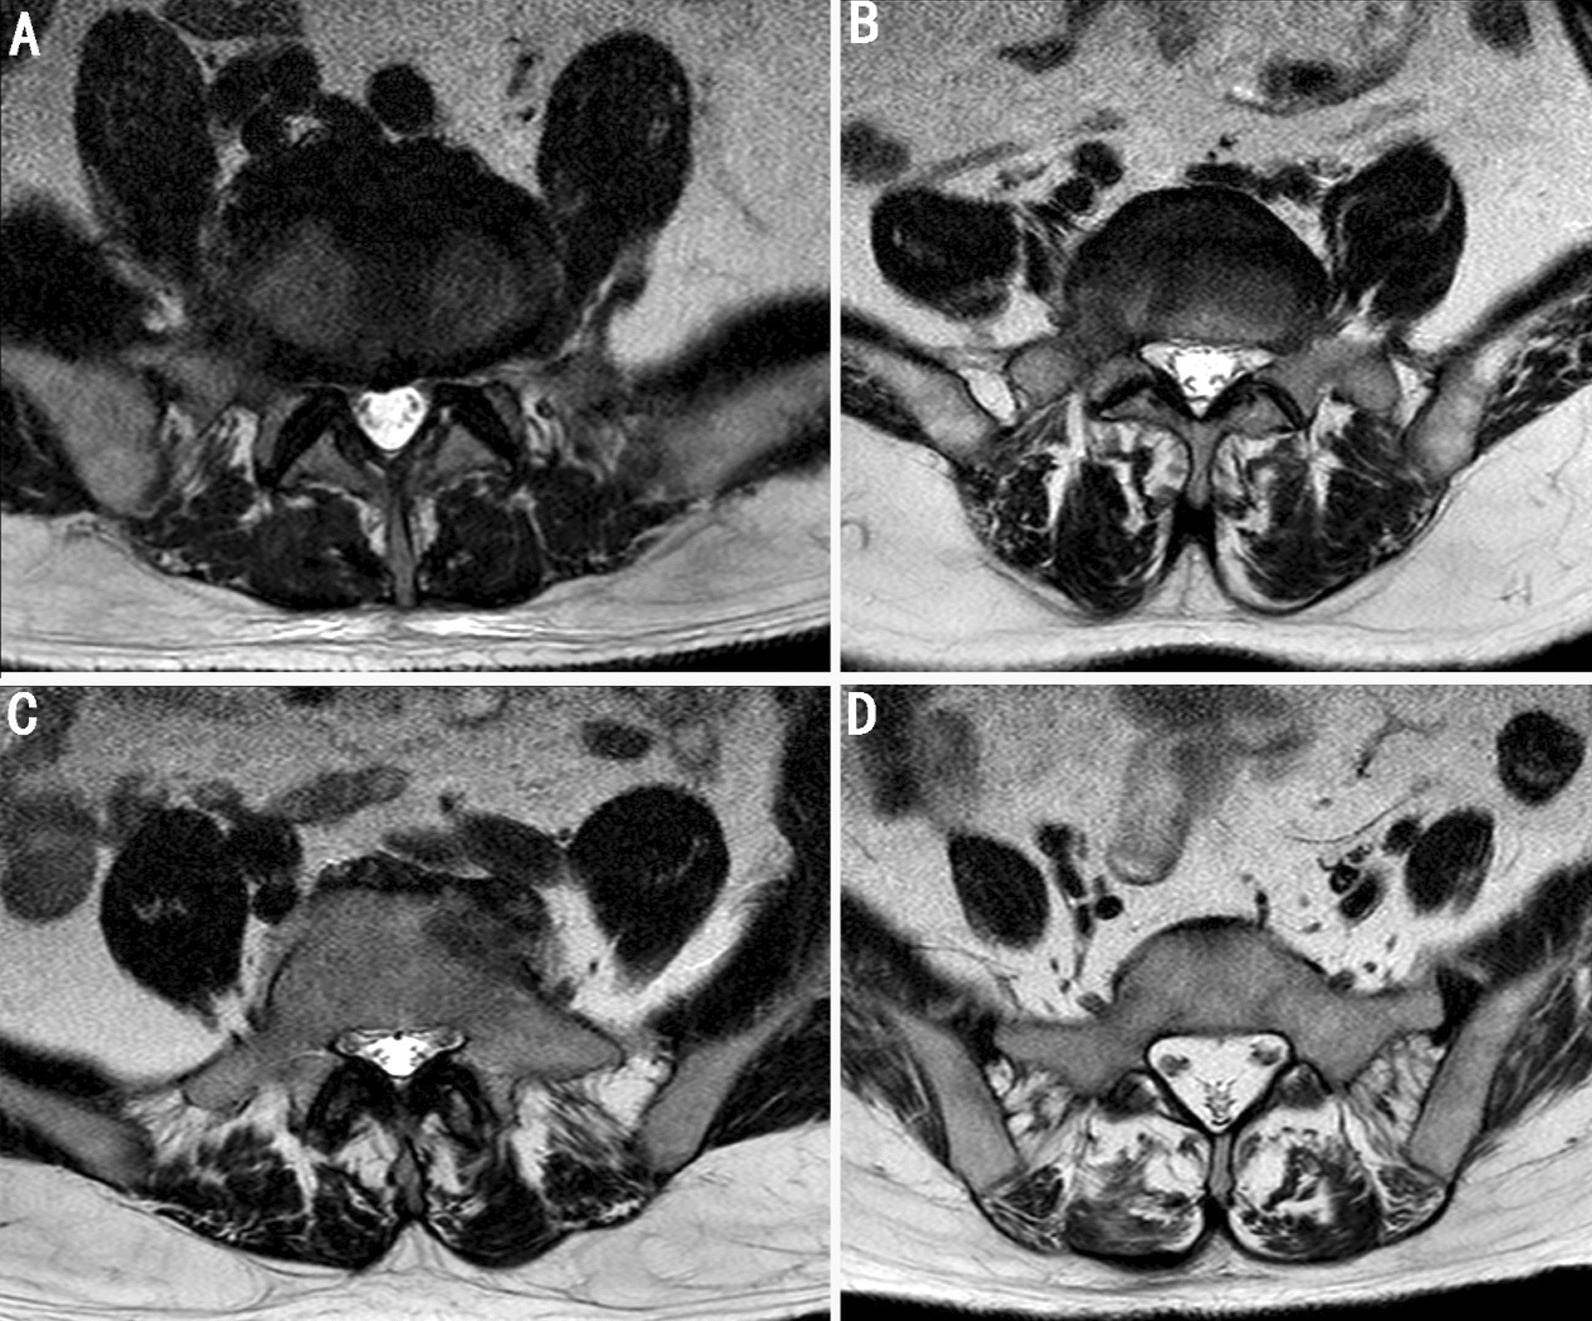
Muscular location and fat infiltration grades on T2 axial MRI slice (evaluation with 4 grade system) A Grade 1 =  < 10% fat infiltration, B Grade 2 = 10–30% fat infiltration, C Grade 3 = 30–50% fat infiltration, D Grade 4 =  > 50% fat infiltration
